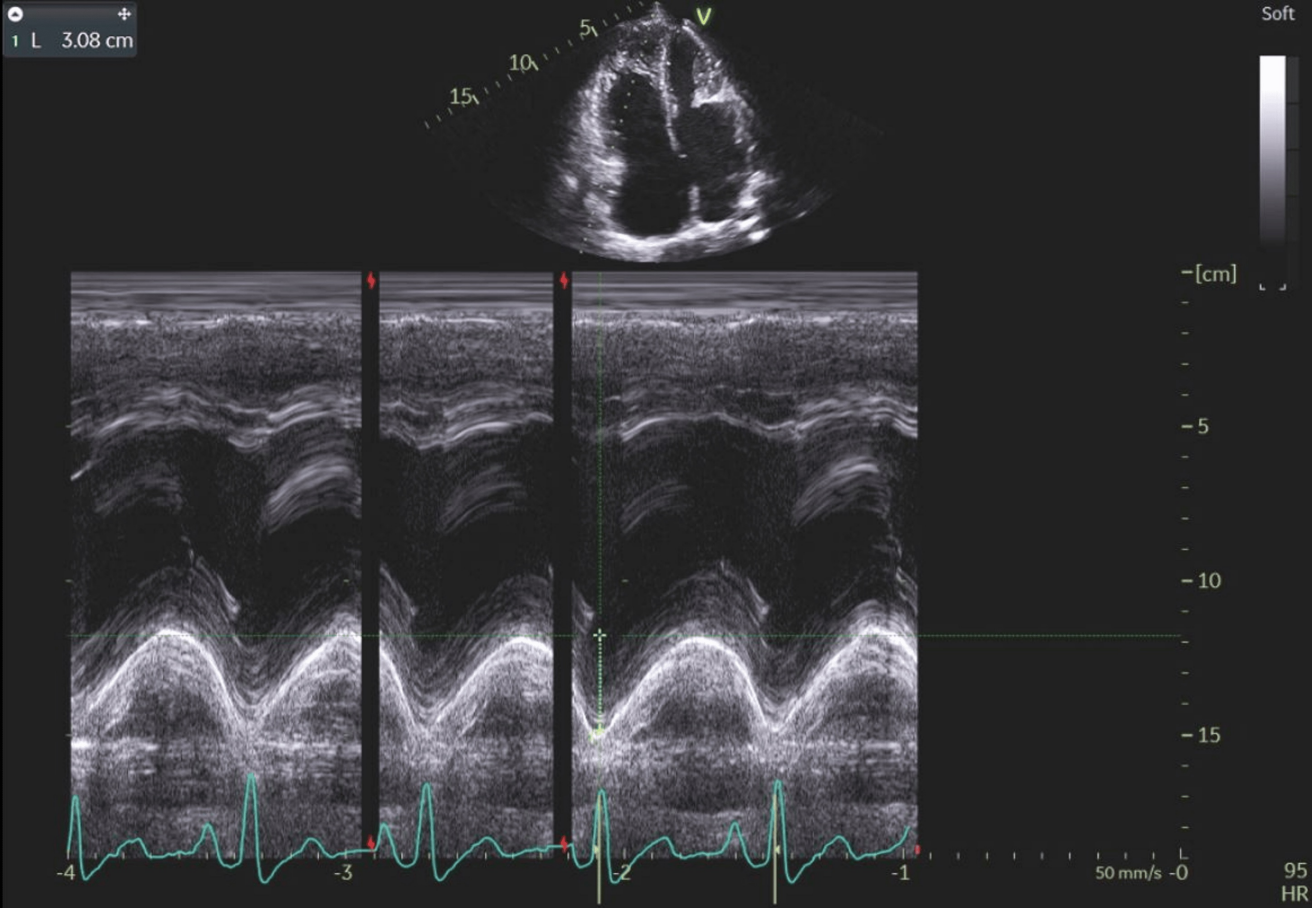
M-mode imaging of the apical four-chamber cross-section showing a TAPSE value of 3.08 cm, which indicates very good right ventricular systolic function and may indirectly suggest volume overload of the right ventricle. TAPSE: tricuspid annular plane systolic excursion
